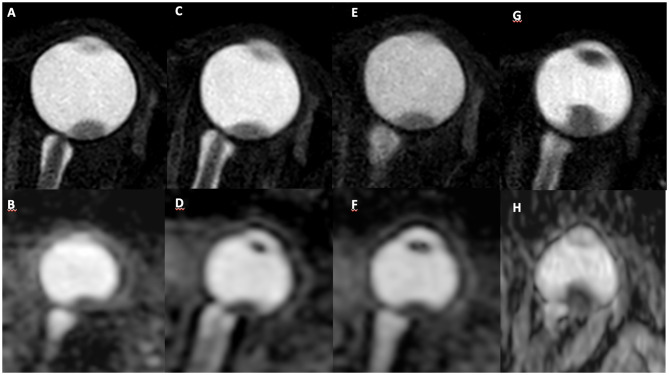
A 59-year-old patient with choroidal melanoma in the left eye (upper temporal quadrant, measuring 9 × 4 mm), was submitted to brachytherapy. Pretreatment MR images: (A) axial T2-weighted sequence and (B) axial ADC map, with mean ADC of 1.241 × 10^−3^ mm^2^/s. MR images collected 3 months after treatment in the same sequences demonstrate stability of lesion size (C) and decreased ADC values (D) (mean ADC: 1.007 × 10^−3^mm^2^/s). MR images 6 months after treatment show stability of the lesion size (E) and ADC values (F). MR images collected 9 months after treatment show an increase in lesion dimensions (G) (13 × 9 mm) and decreased ADC values (H) (mean ADC: 0.794 × 10^−3^mm^2^/s).
